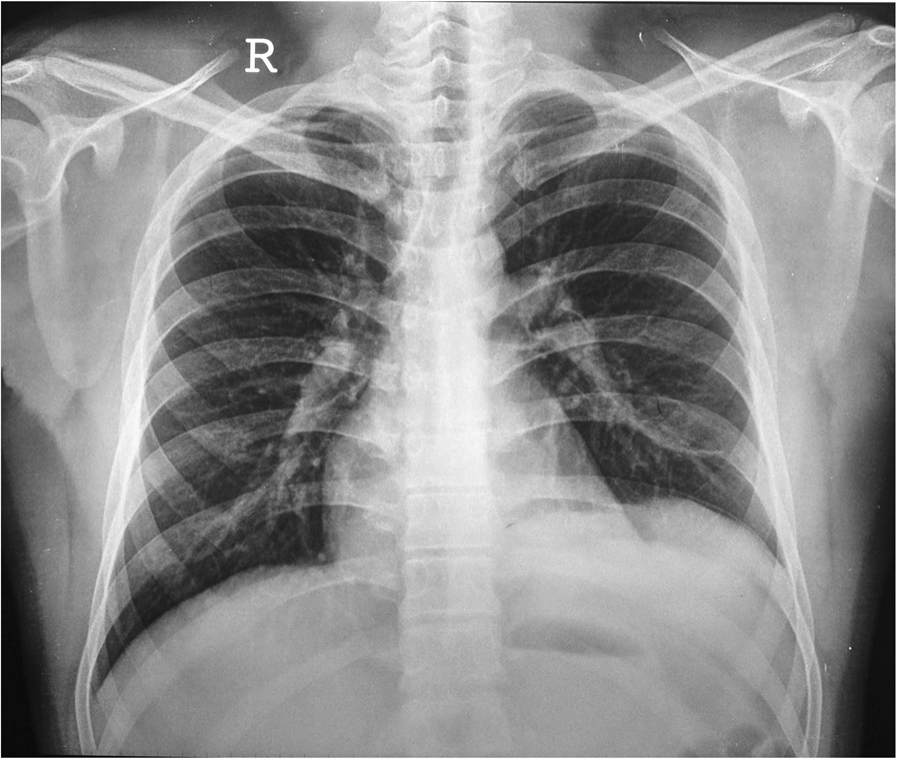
X-ray chest showing raised left dome of the diaphragm
